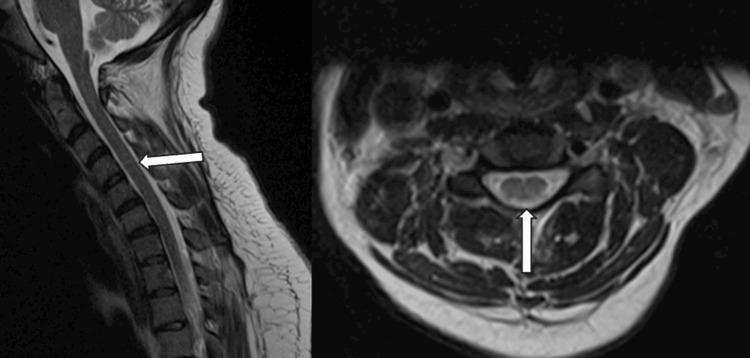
MRI of the cervical spine sagittal view (left), axial view (right) showing multiple degenerative discs. No abnormal enhancements were observed.
